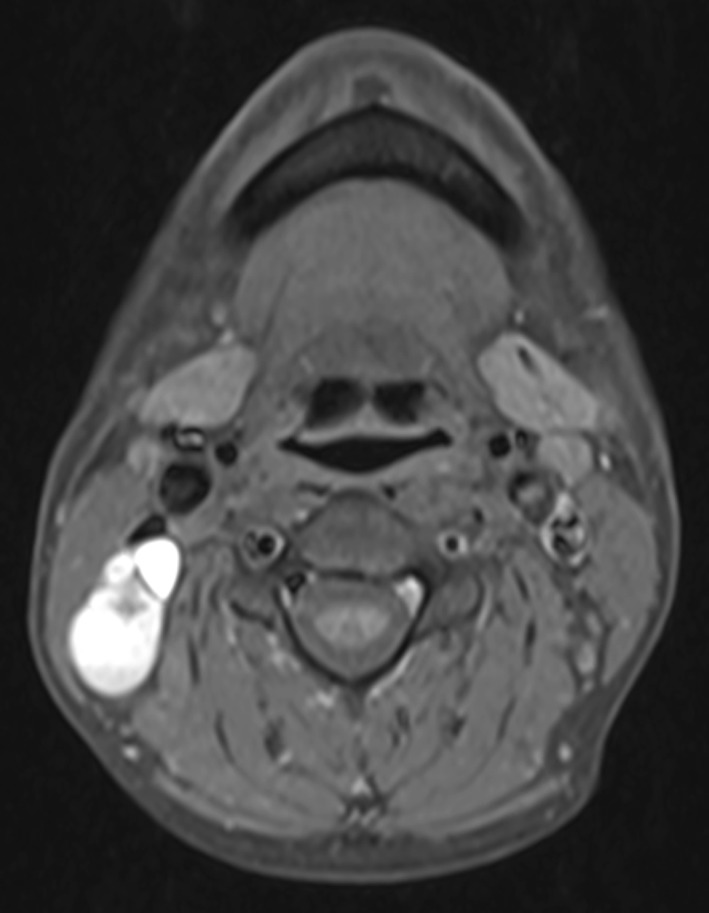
Fused, metastatic T1 hyperintense Level II lymph nodes. The minimal diameter of the largest node is 16 mm. Contrary to CT or US, MRI can identify increased T1 signal intensity indicating thyroglobulin
